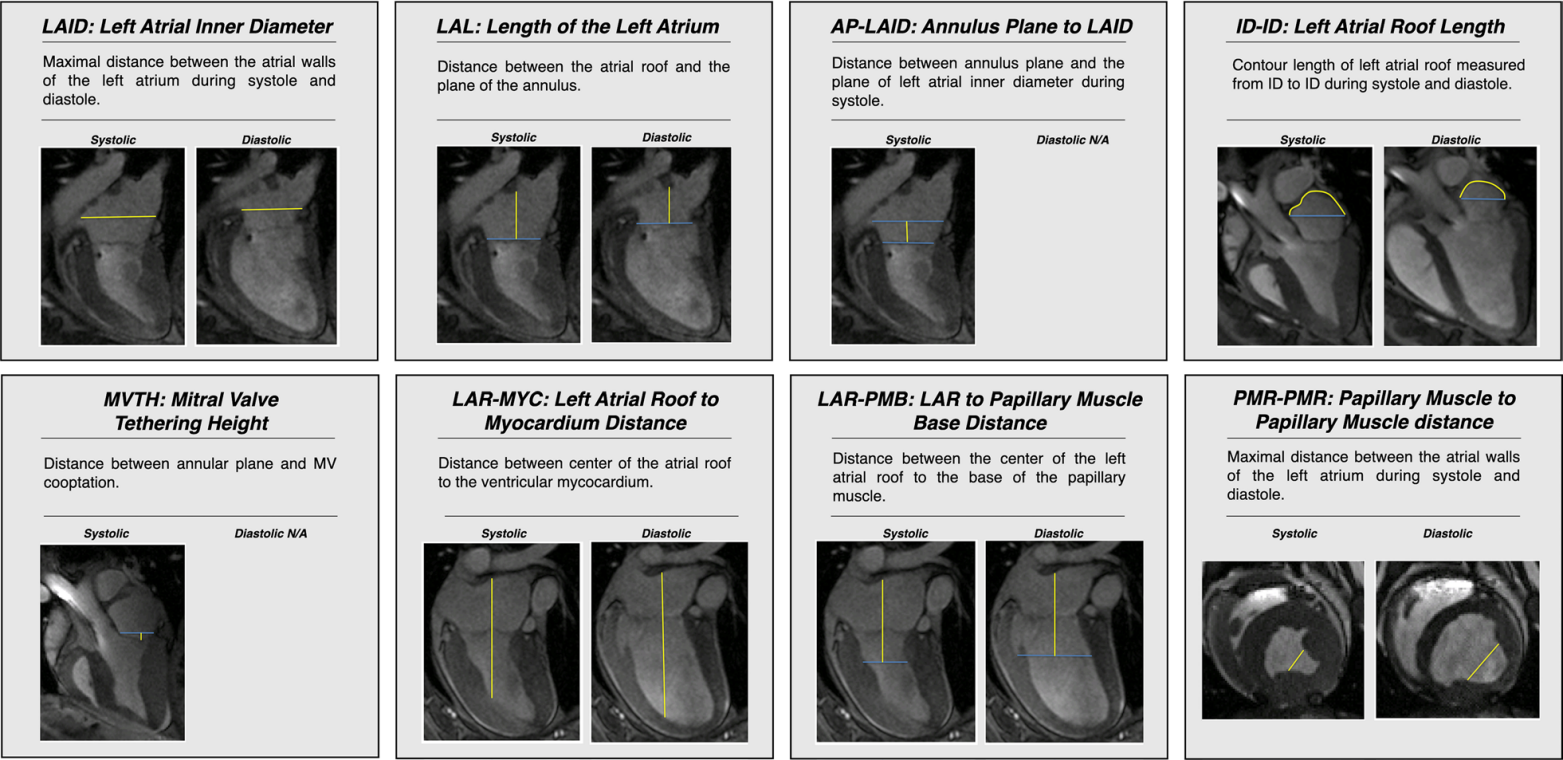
MRI parameters measured at baseline and 8 weeks post myocardial infarction
